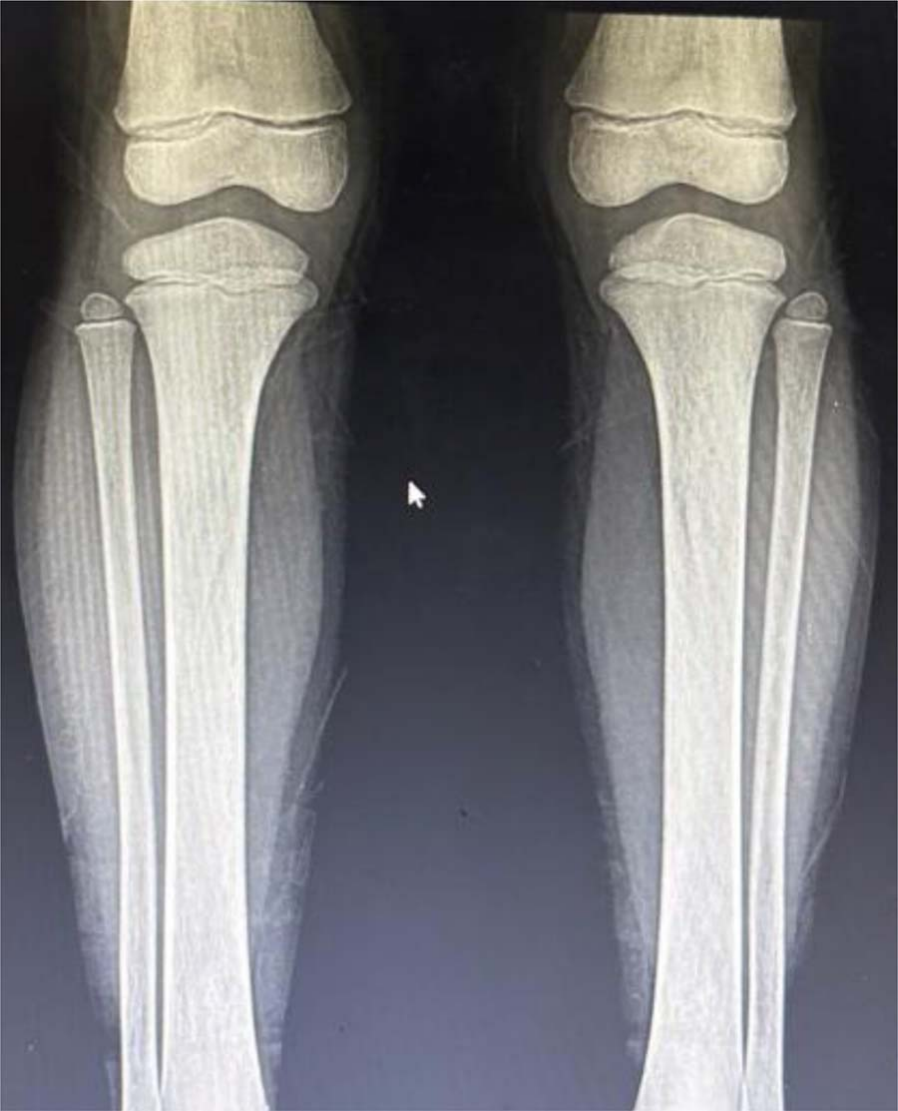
Lower extremity long bone involvement in LCH. Anteroposterior radiograph of both lower extremities revealing multiple bilateral osteolytic lesions in the femoral shafts and metaphyses. The lesions display a geographic pattern with well-defined, irregular borders and a narrow zone of transition. Cortical integrity is preserved, and no periosteal reaction is observed. These findings are consistent with chronic skeletal involvement in Langerhans cell histiocytosis.
